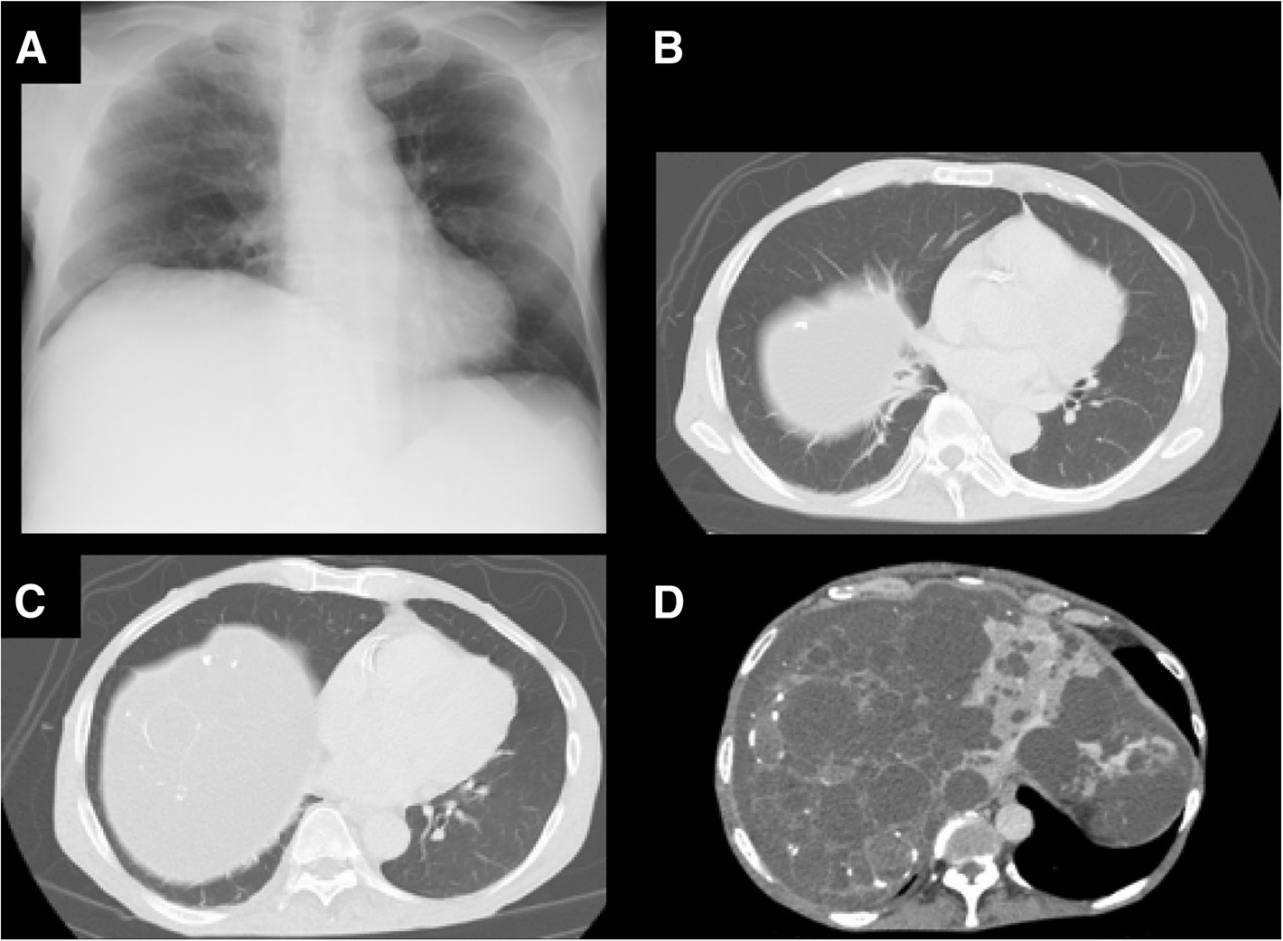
Preoperative chest X-ray and computed tomographic (CT) images of a 66-year-old man with multiple liver cysts. a Chest X-ray showed an elevation of right diaphragm. b–d CT showed hepatomegaly with multiple liver cysts and no cystic legions in the bottom of both sides of lung
